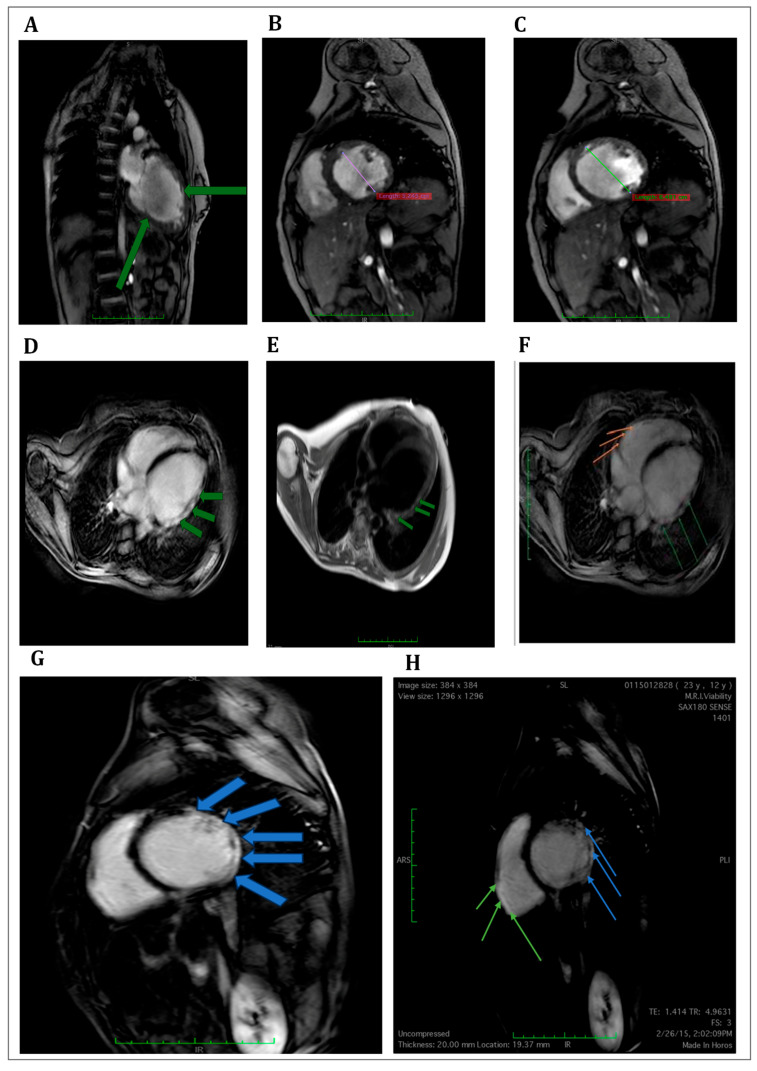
Cardiac MRI (KU-48.IV-9). (A) Marked left ventricular dilatation (green arrow). (B) End-systolic LV diameter of 5.24 cm (red line). (C) End-diastolic LV diameter of 6.48 cm (green line). (D) Subepicardial late gadolinium enhancement in the lateral LV wall on axial imaging. (E) Dark-blood axial imaging showing fatty infiltration in the same region of the lateral LV wall. (F) Axial images showing late gadolinium enhancement at the lateral wall of the left ventricle (green arrows) and involvement of the right ventricular wall (orange arrows) indicating fibrotic changes. (G) Two-chamber view: Late gadolinium enhancement at the lateral wall of the left ventricle (blue arrows) indicating fibrotic changes. (H) Two-chamber view: Late gadolinium enhancement at the anterior, lateral, and inferior walls of the left ventricle (green arrows) and involvement of the right ventricular wall (green arrows) indicating fibrotic changes.
